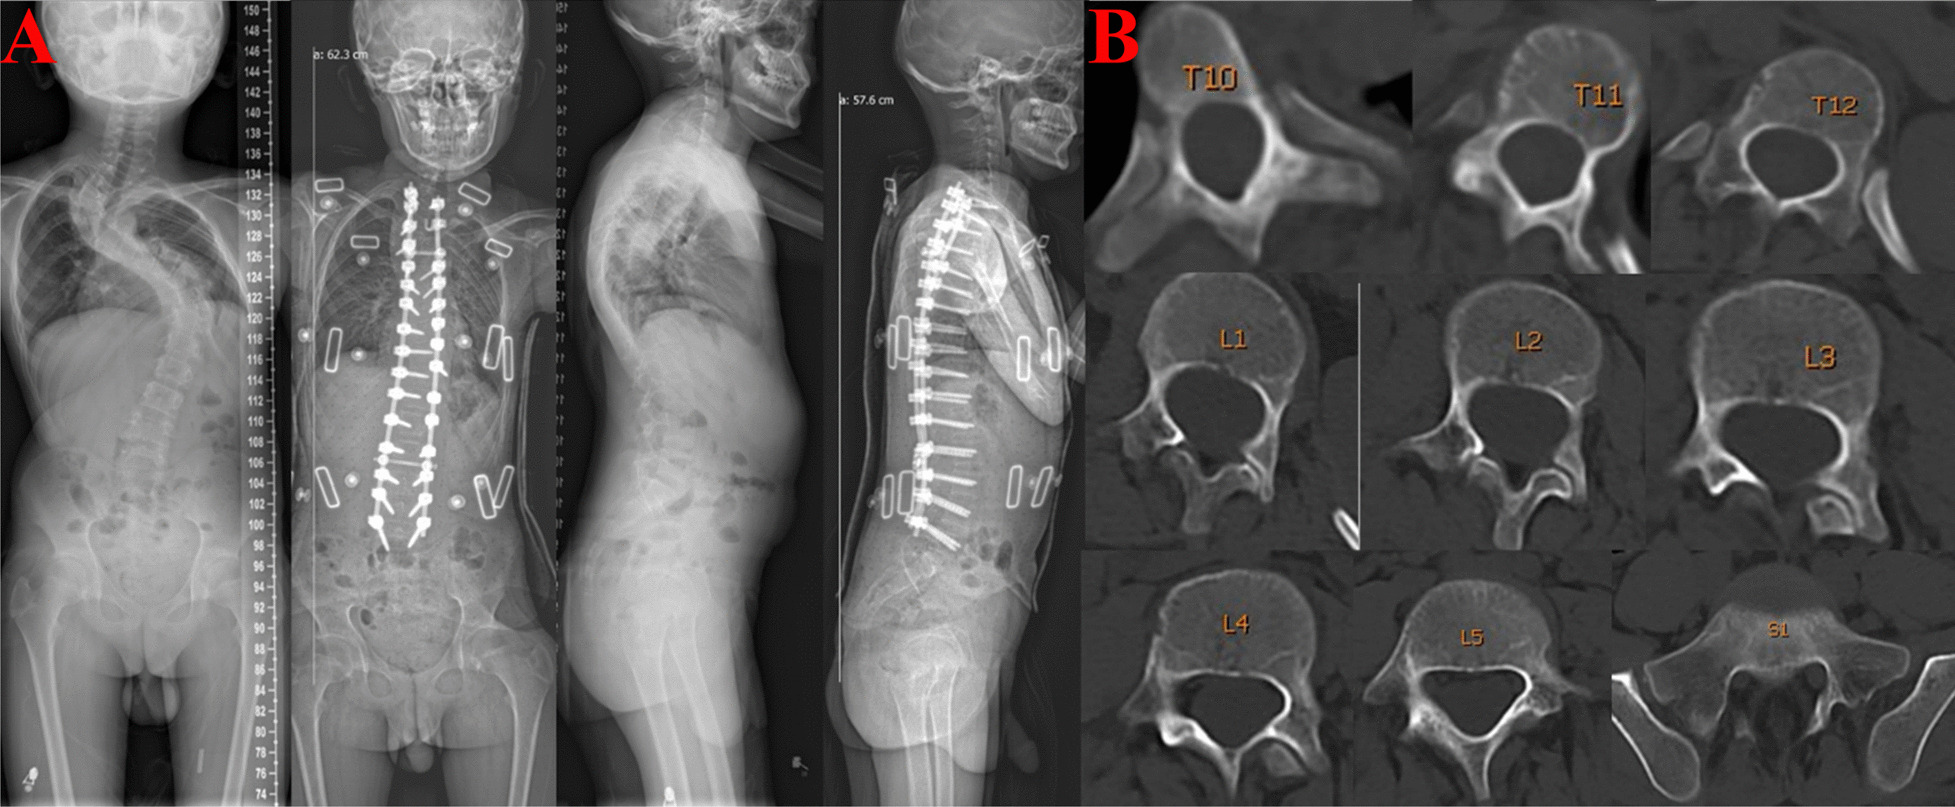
Representative preoperative and immediate postoperative images for neurofibromatosis type 1 (NF-1) scoliosis. A The 13-year-old patient obtained significantly improvement both in coronal and sagittal spinal deformity after undergoing posterior correction with t-EMG and O-arm-assisted pedicle screw fixation. B Routine preoperative CT indicated extremely thin pedicles in apical region
